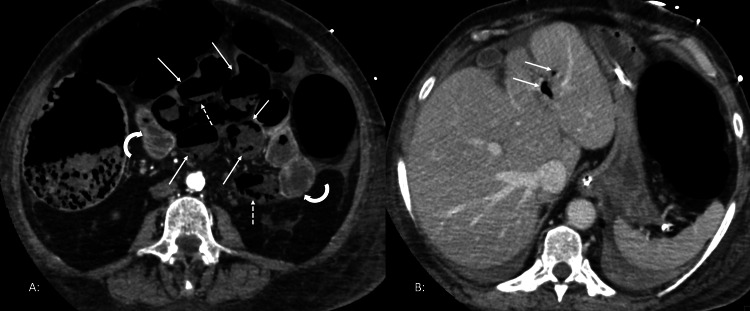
CT scans of the abdomen and pelvis with contrast showing findings of small bowel ischemia. (A) Multiple dilated loops of small bowel in the central abdomen, non-enhancement of the bowel wall (solid arrows) compared to normally enhancing adjacent bowel loops (curved arrows). There is also evidence of air in the bowel wall or pneumatosis intestinalis (dashed arrows); (B) Air within the portal venous radicals in the left hepatic lobe (solid arrows)
